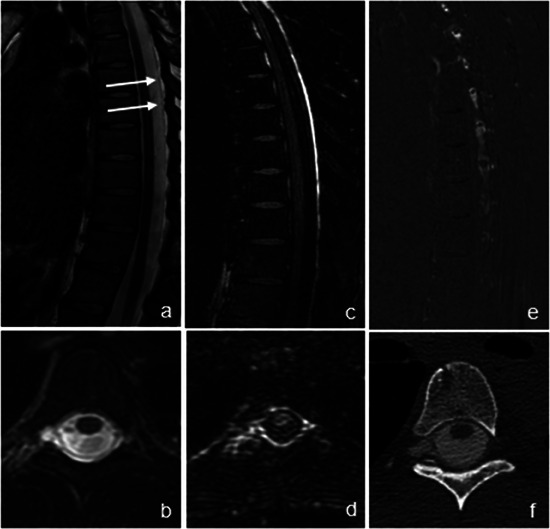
Presentation of a case in which the cerebrospinal fluid (CSF) lamella is clearly visualized on both intravenous contrast‐enhanced heavily T2‐weighted fluid‐attenuated inversion recovery (ceHT2‐FLAIR) MRI and noncontrast MR myelography. A 32‐year‐old female patient with intracranial hypotension (case #4) presented with therapy‐resistant, position‐dependent headache without any history of trauma. On noncontrast MRI, the modified DIXON T2 sequence revealed a right lateral and dorsal epidural fluid lamella in the sagittal plane (white arrows) (a), which was confirmed in the axial plane (b). On ceHT2‐FLAIR, the epidural CSF lamella is clearly visible despite motion artifacts and demonstrates strong contrast enhancement in both sagittal and axial planes immediately postinjection (c, d), again with right lateral predominance in the axial view (d) and the right paramedian subtraction image (e). CT myelography (f) confirmed the presence of the CSF lamella with right lateral predominance; however, a causative osteophyte could not be identified. The patient therefore received a nontargeted epidural blood patch. In this case, ceHT2‐FLAIR independently confirmed the findings of noncontrast MR myelography.
